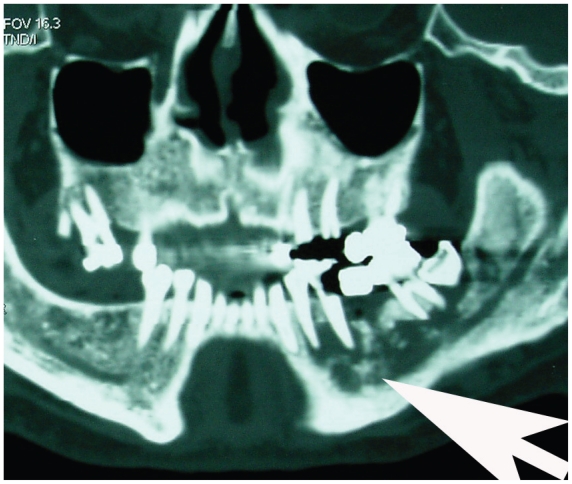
Computed tomography showing a wide osteolytic area in the left part of the mandible.
